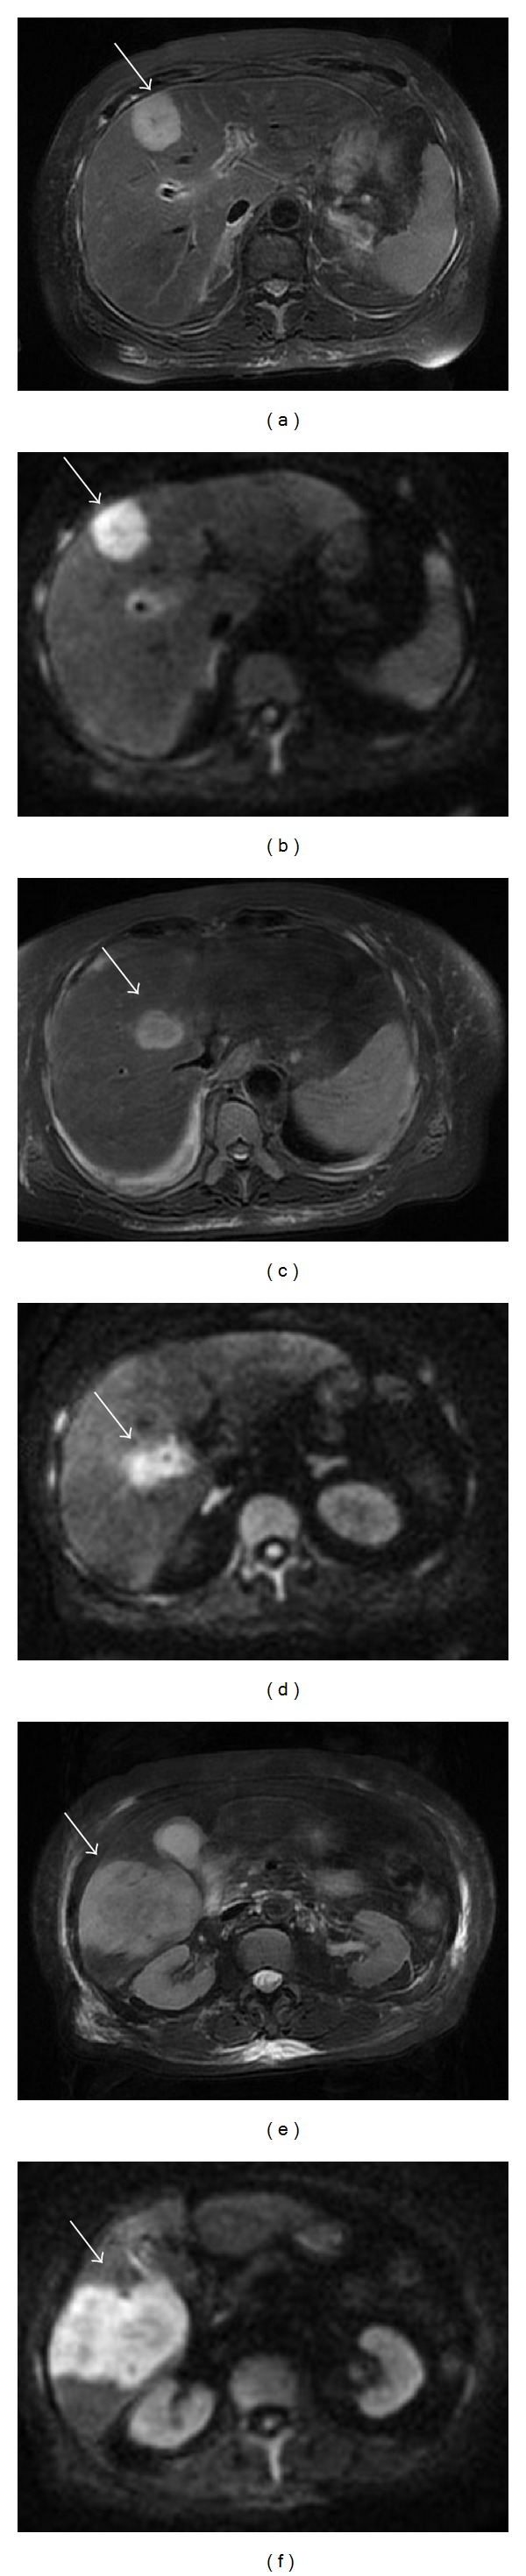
Noncontrast abdominal magnetic resonance imaging. Masses, 3.2 cm, 7 cm, and 2.2 cm in size, were revealed at segments 5, 6, and 8, respectively ((a)–(f)). These masses exhibited high signal intensity on T2- and diffusion-weighted imaging. Penetrating vessels were evident among the masses, suggesting hepatic lymphoma.
